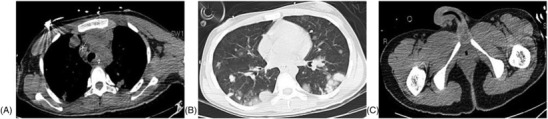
Total body CT scan. (A and B) The mediastinal masses and multiple nodules in both lungs did not shrink significantly. (C) Pelvis CT showed no abnormal mass shadow as before.
